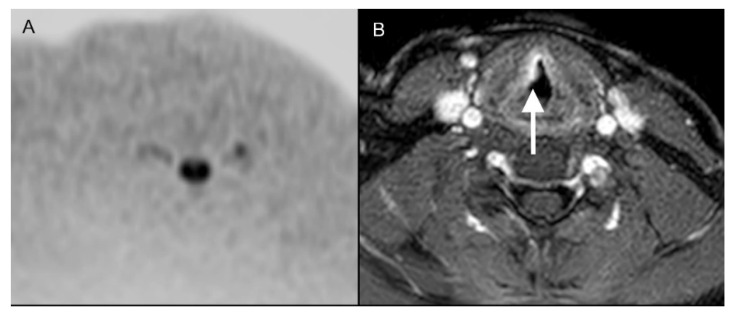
(A) A 37-year-old patient with a pT1a cN0 cM0 squamous cell carcinoma of the right vocal cord, which was not detectable in DWIBS (A) but was correctly diagnosed in contrast-enhanced transversal T1-weighted scans with fat saturation ((B); white arrow) by both readers.
